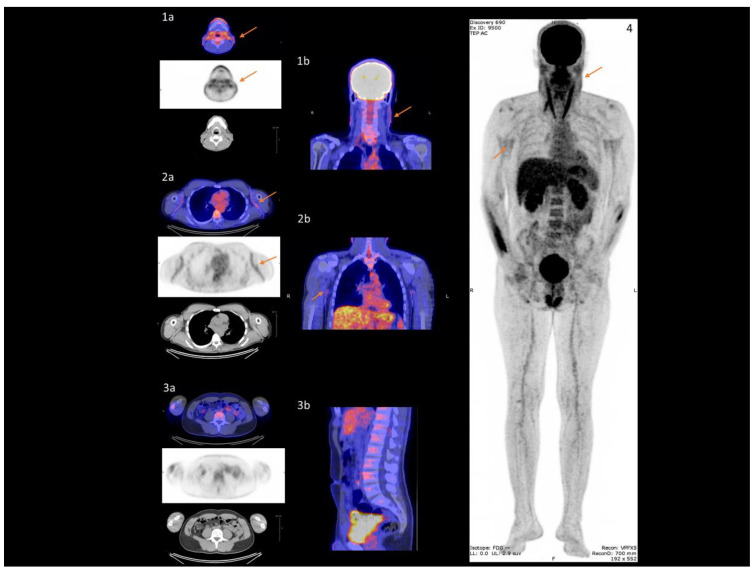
18F-FDG uptake in specific damaged skin in PXE. Damaged skin in neck (arrows (1a,1b)) and axillary folds (arrows (2a,2b)) vs healthy skin in lumbar region (3a,3b) on PET/CT imaging. Maximum intensity projection indicates damaged skin sites (arrow 4).
